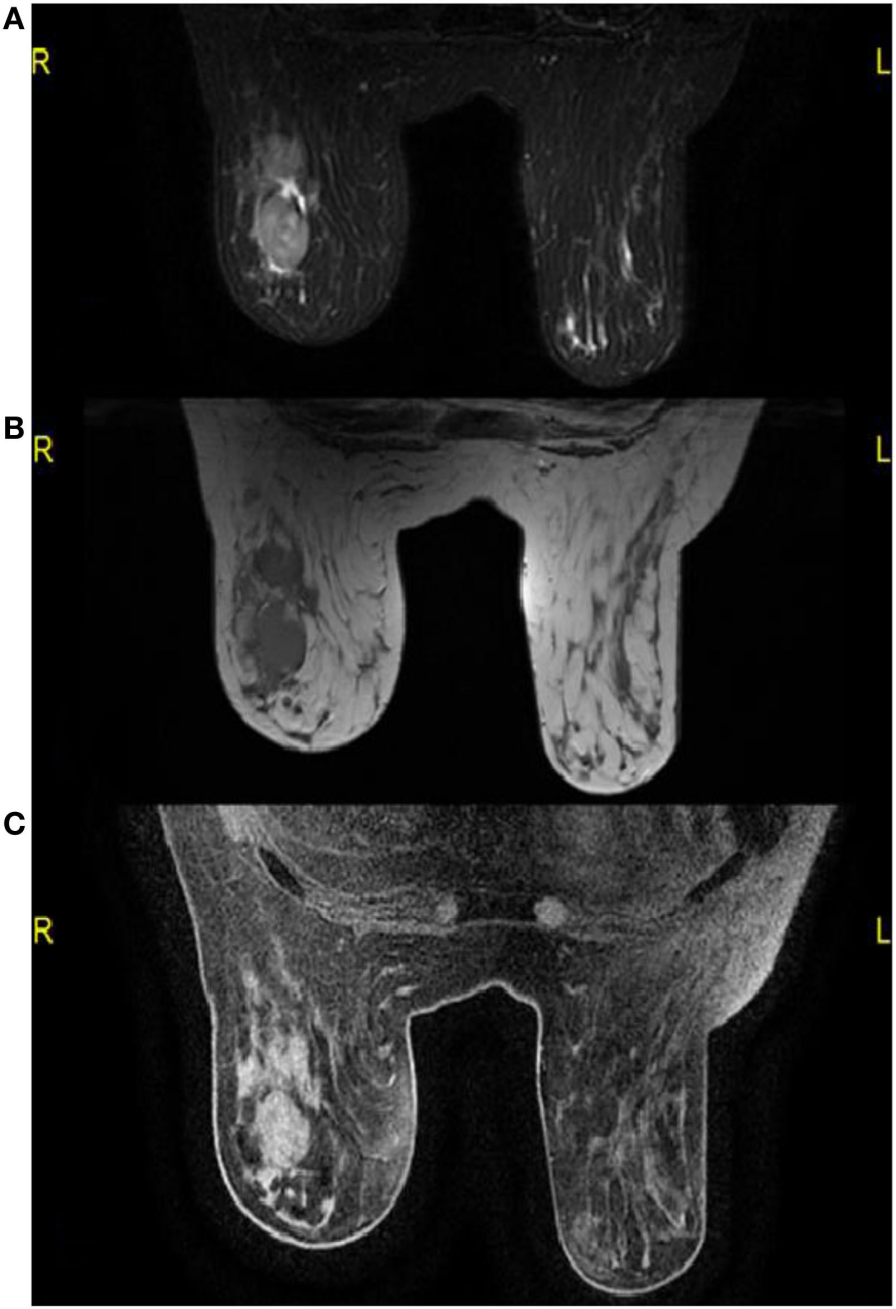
On MRI, multiple round masses are localized in the outer upper quadrant of the right breast, which present a hyperintense signal on T2-weighted images and fat saturation (A) and a hypointense signal on T1-weighted images (B). These lesions present a faint contrast enhancement on gadolinium-enhanced T1-weighted images with fat saturation (C).
